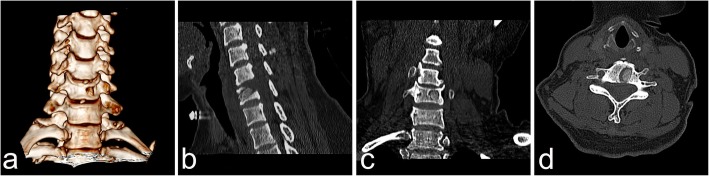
CT images of the channel immediately postoperatively, including the axial plane view (a), coronal reconstruction view (b), sagittal reconstruction view (c), and three-dimensional view (d), showed the trajectory of the channel. No migration of the repaired bone occurred
